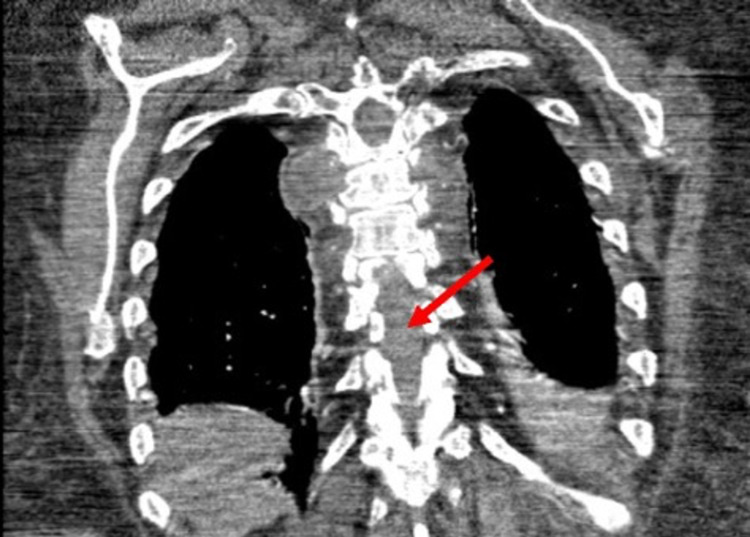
The CT scan showed a paravertebral soft tissue mass at the T6-T7 level with the destruction of part of the T6 vertebral body
